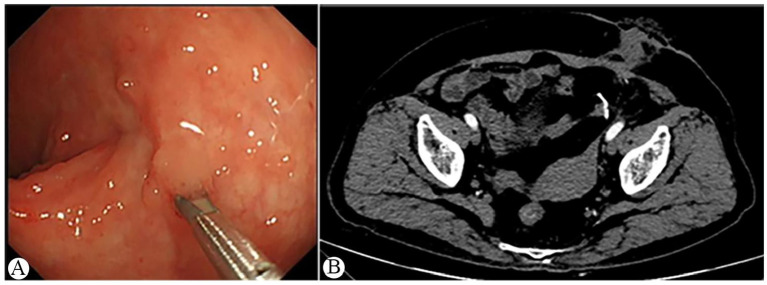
Final follow-up colonoscopic and CT findings. (A) Repeat colonoscopy at 115 days after polypectomy showing well-healed intestinal mucosa with a residual titanium clip; (B) Follow-up CT scan confirming complete resolution of the previously identified rectal wall abscess.
